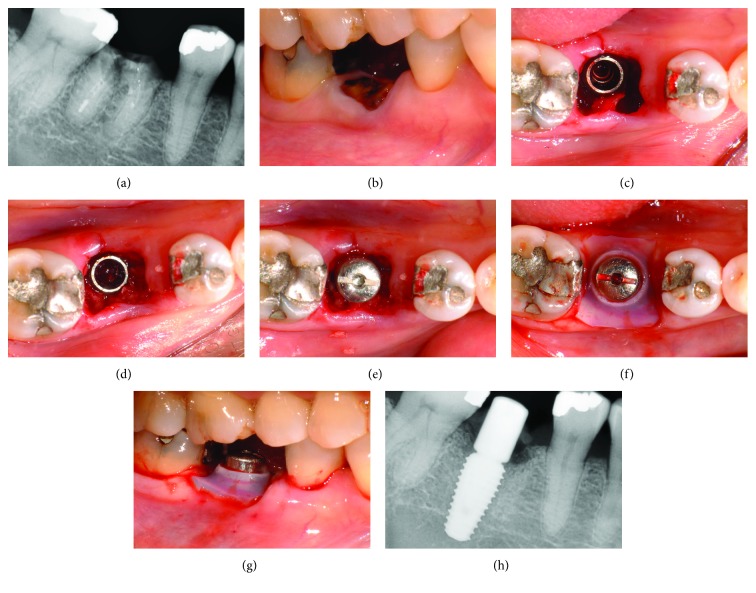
(a) Periapical radiograph of tooth 46 from the initial examination. (b) Buccal view of tooth 46 at the initial examination. (c) Implant inserted into interradicular septum. (d) Clinical condition after gap filling with autologous particulate bone. (e) Clinical condition after the installation of a cylinder-type healing abutment. (f) Occlusal view after the application of silicone cap on healing abutment. (g) Buccal view after the application of silicone cap on healing abutment. (h) Periapical radiograph immediately after surgery.
